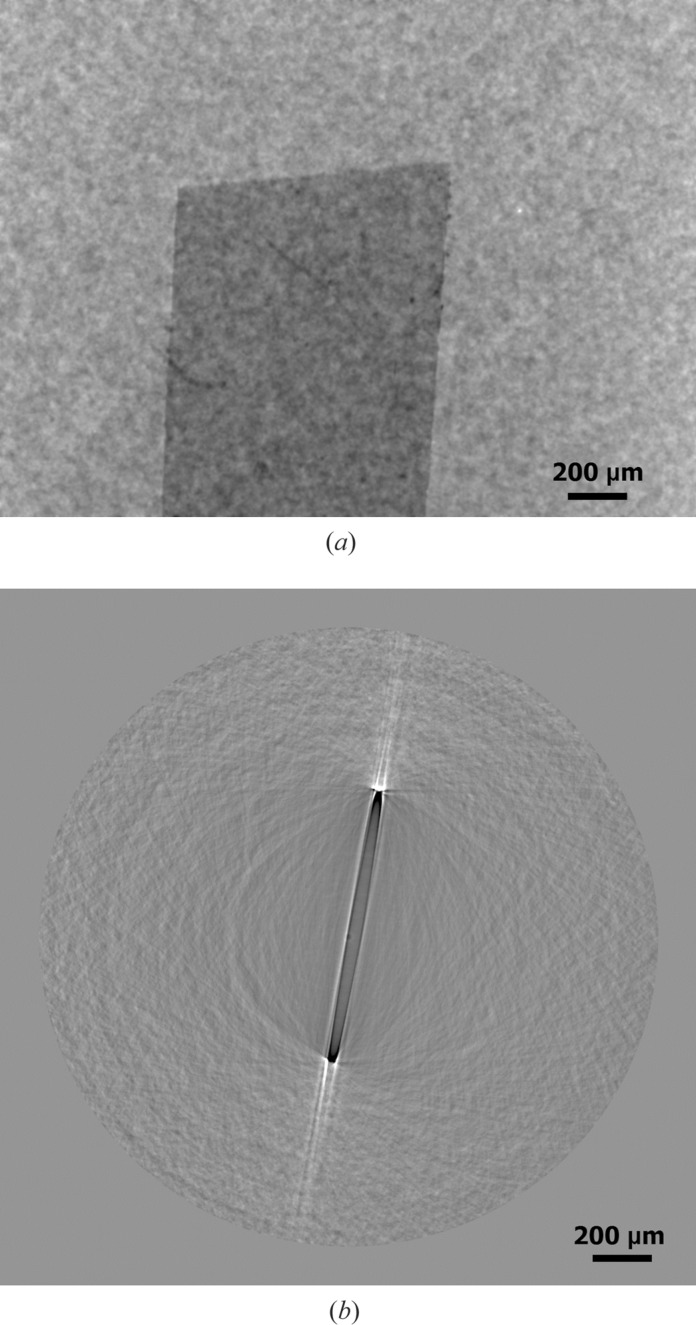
(*a*) Phase map resulting from *ANKAphase* application and (*b*) CT slice as a result of CT reconstruction of phase map projections.
